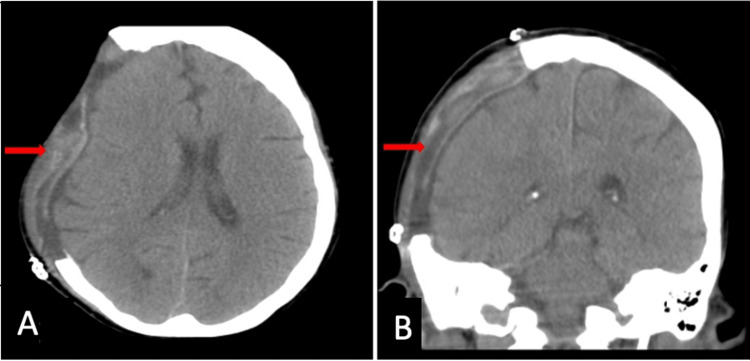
Non-contrast CT head post right hemicraniectomy (red arrows indicate the removal of bone flap and evacuated SDH) SDH: Subdural hematoma, A: Axial view, B: Coronal view
